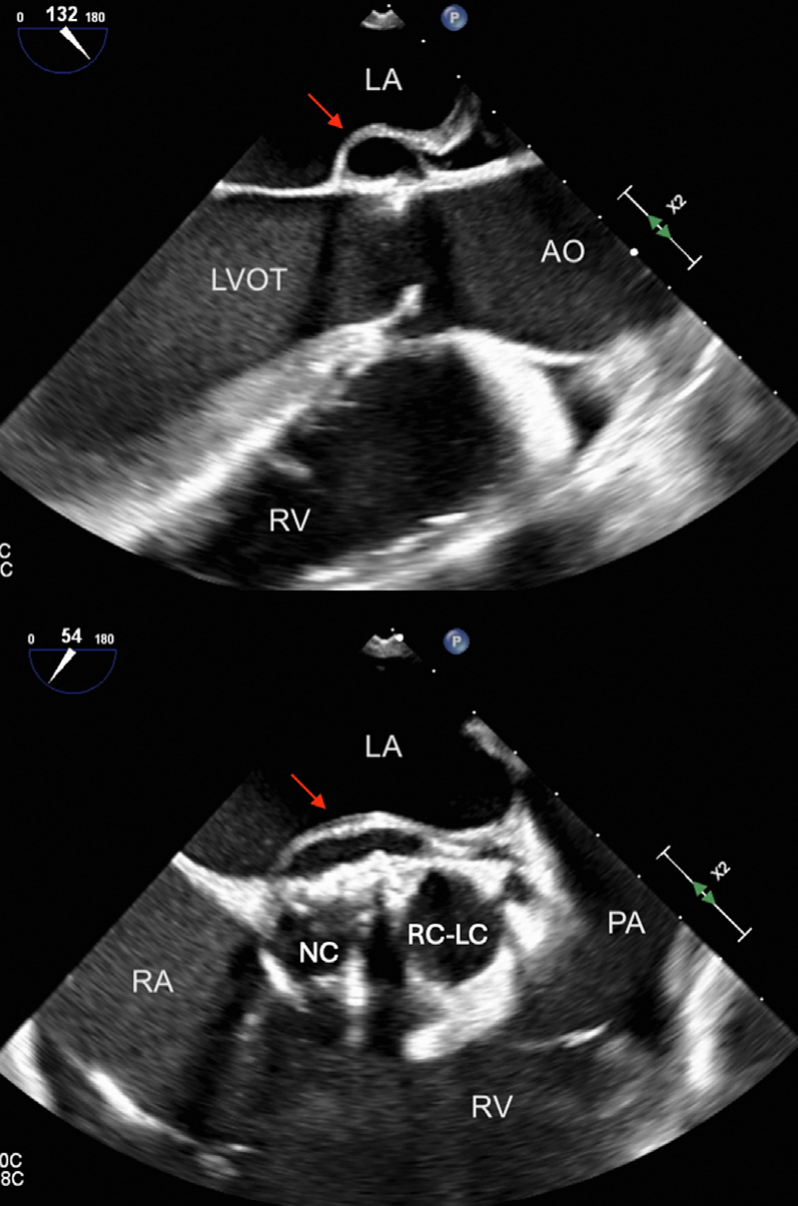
Intraoperative Transesophageal Echocardiogram Intraoperative transesophageal echocardiogram showing BAV with RC-LC fusion with vegetation, NC, and pseudoaneurysm (red arrows). PA = pulmonary artery; other abbreviations as in Figures 2 and 3.
